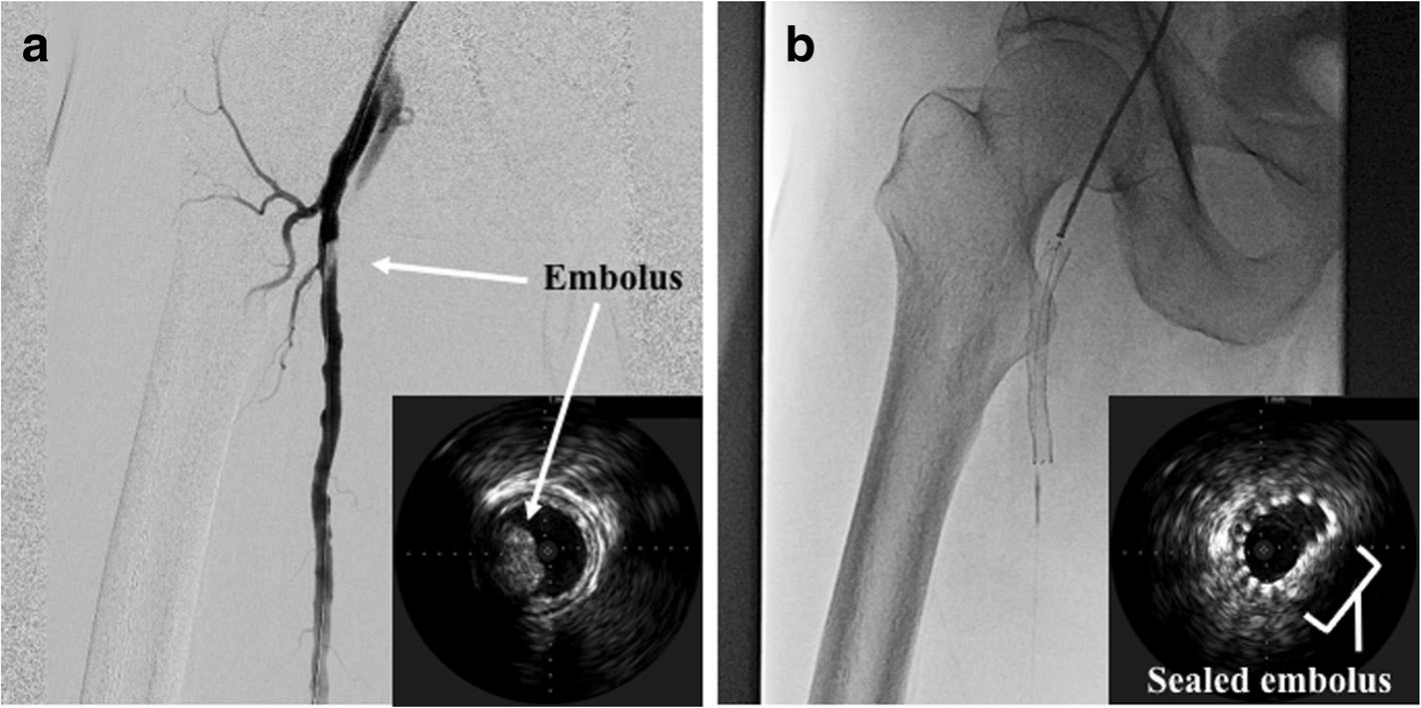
a We confirmed the embolus (arrows) was attached to the proximal superficial femoral artery by angiography and intravascular ultrasound. b We implanted a 7.0 × 60-mm self-expandable nitinol stent to seal the embolus (square bracket)
